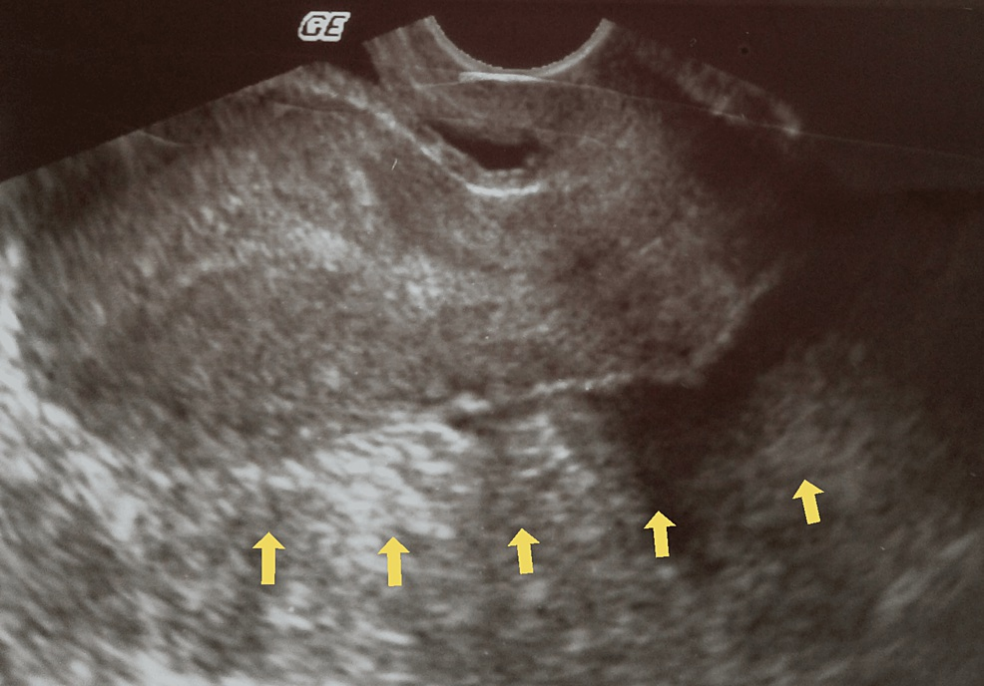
Transvaginal ultrasound imaging of ectopic ovarian pregnancy (our case) The presence of free fluid and blood clots in the cul-de-sac (yellow arrows) and the absence of an intrauterine gestational sac support the diagnosis of ruptured ectopic pregnancy.
